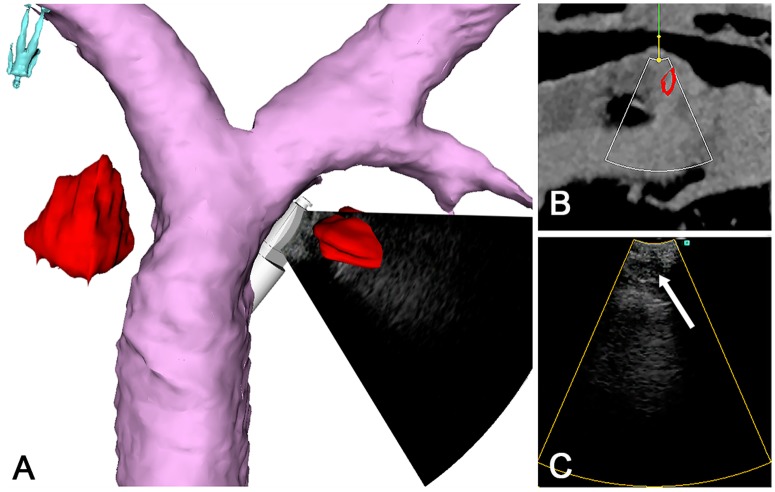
Graphical user interface, patient 4. (A) The CT models of the central airways, a 4R and a 4L lymph node target, the tip of the EBUS-scope and the real-time EBUS image. (B) The corresponding image plane from preoperative CT. The thin white sector represents the exact position of the EBUS image. (C) Regular, real-time EBUS image, showing the 4R lymph node (arrow).
